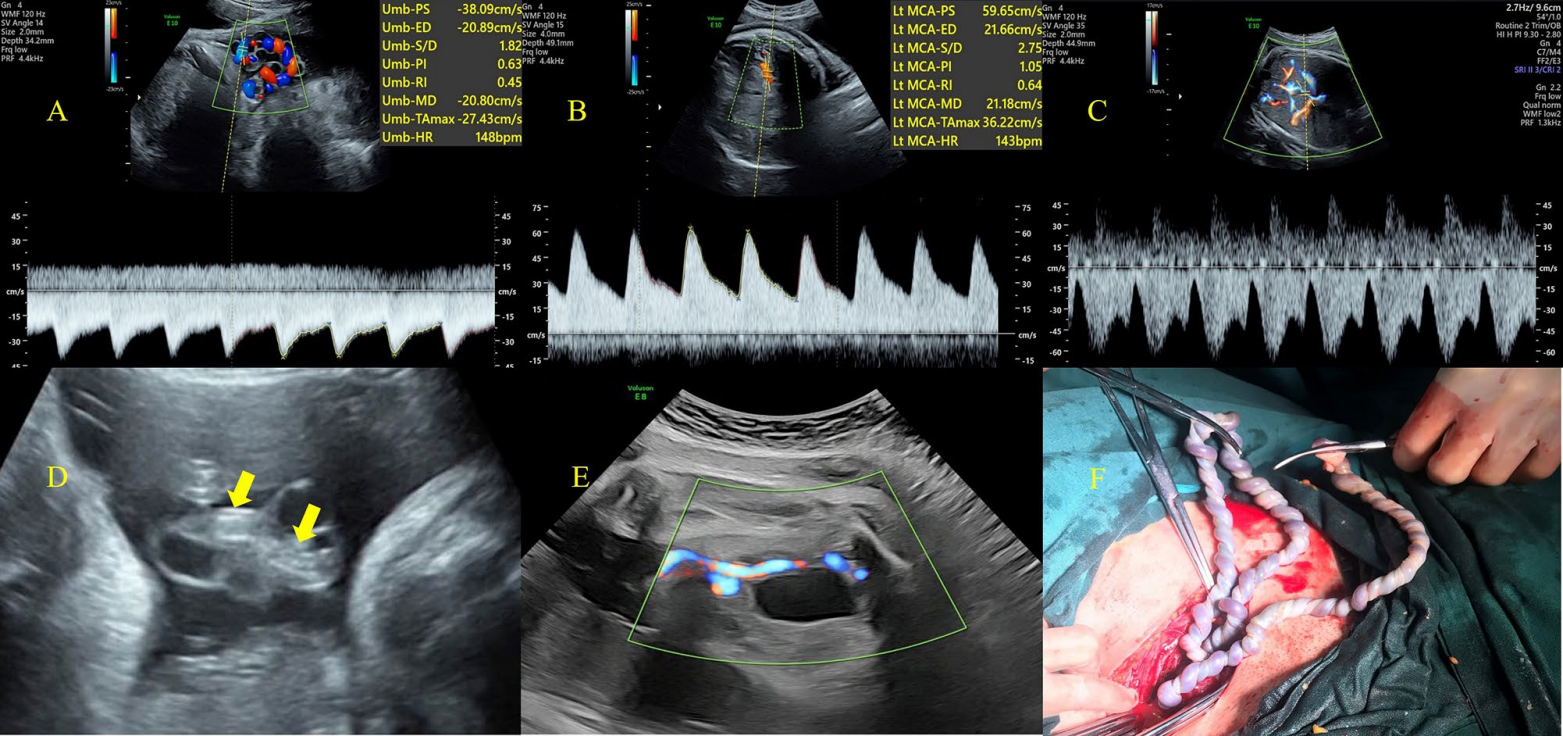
Hemodynamics arrangement of the UAT fetuses (GA at diagnosis: 36^1/7^ weeks). A Waveforms from UA, the value of S/D and PI were both abnormal (<5th centile for gestational age). B Waveforms from MCA, the value of PI was abnormal (<5th centile for gestational age). C Ductus venosus Doppler recording showed the ‘a-wave’, which was close to the zero line. D, Hyperechoic thromboembolism (yellow arrow) was observed in a part of the UA through two-dimensional ultrasound. E Colour Doppler flow showed only one umbilical artery in the section of bladder. F Excessive torsion of umbilical cord presented by gross observation
